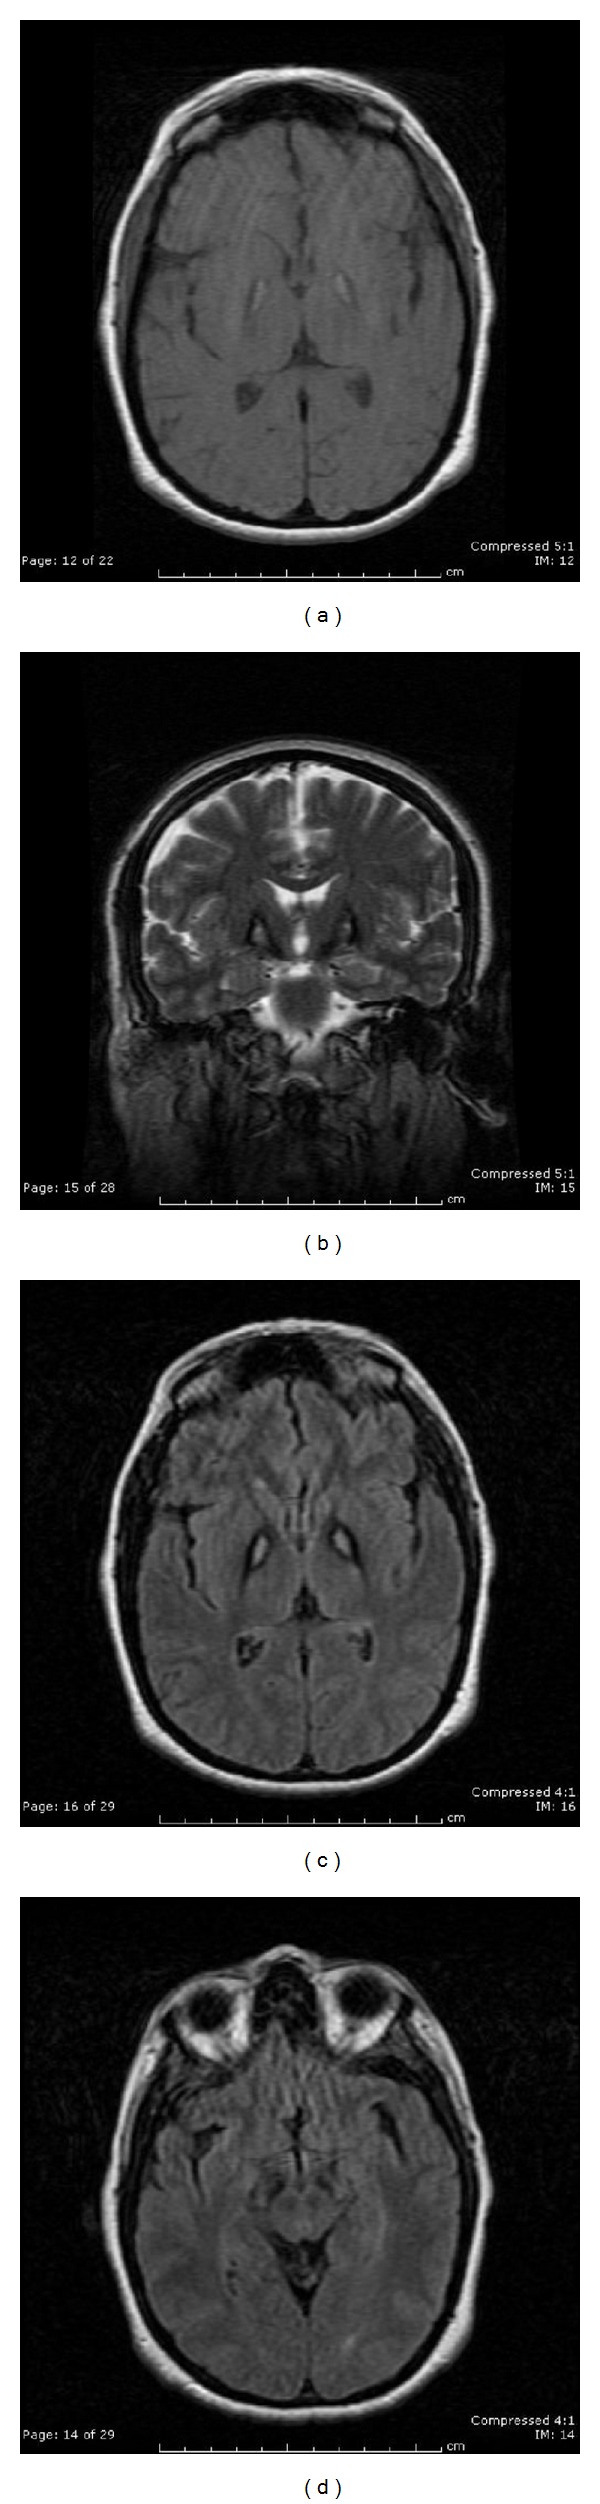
(a) Axial T1 weighted, (b) coronal T2 weighted, and (c) axial fluid attenuation inversion recovery MRI showing low signal intensity in the bilateral globus pallidus with a medial area of signal intensity, presenting the classic “eye-of-the-tiger” sign. (d) Axial fluid attenuation inversion recovery MRI shows bilateral low signal intensity in the substantia nigra pars reticulata.
